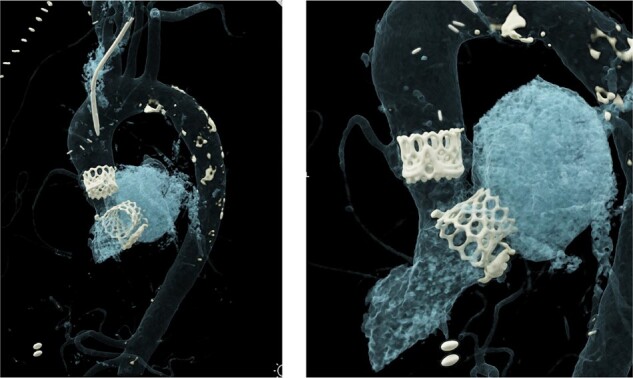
Pre-operative CT evaluation reveals high risk for LVOT obstruction. Cardiac CT shows an angle 112° between LV inflow and outflow. Septal thickness is 7 mm (basal) and 14 mm (midventricular). LVOT diameter is 20 × 27 mm.
